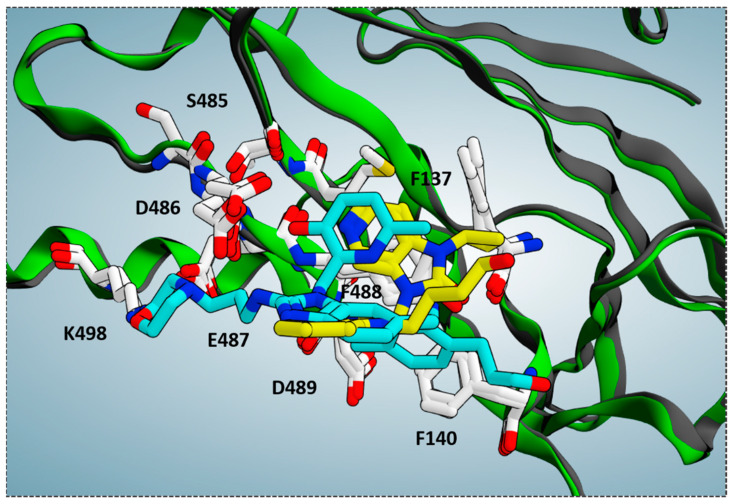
Superimposition of the X-ray complexes of the RSV F protein in presence of the two inhibitors TMC-353121 (C atom in cyan; pdb code = 5EA5) [13] and BMS-433771 (C atom in yellow; pdb code = 5EA7) [13].
